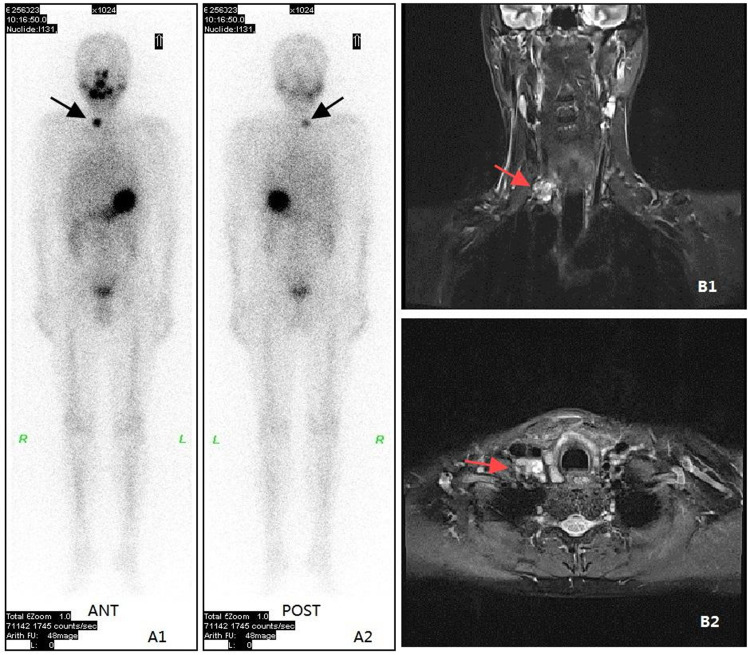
MRI features of lymph nodes positive on ^131^I-Rx-WBS. (A1, A2) anterior and posterior ^131^I-Rx-WBS images showing a positive lymph node in the right cervical level IV (black arrow), suggestive of cervical lymph-node metastasis from thyroid carcinoma. (B1, B2) coronal and axial fat-suppressed T2-weighted cervical MRI of the same patient; the corresponding positive node in right level IV is irregular in shape, exhibits heterogeneous hyper-intense T2 signal, lies adjacent to a vessel, and shows an indistinct hilum (red arrow).
